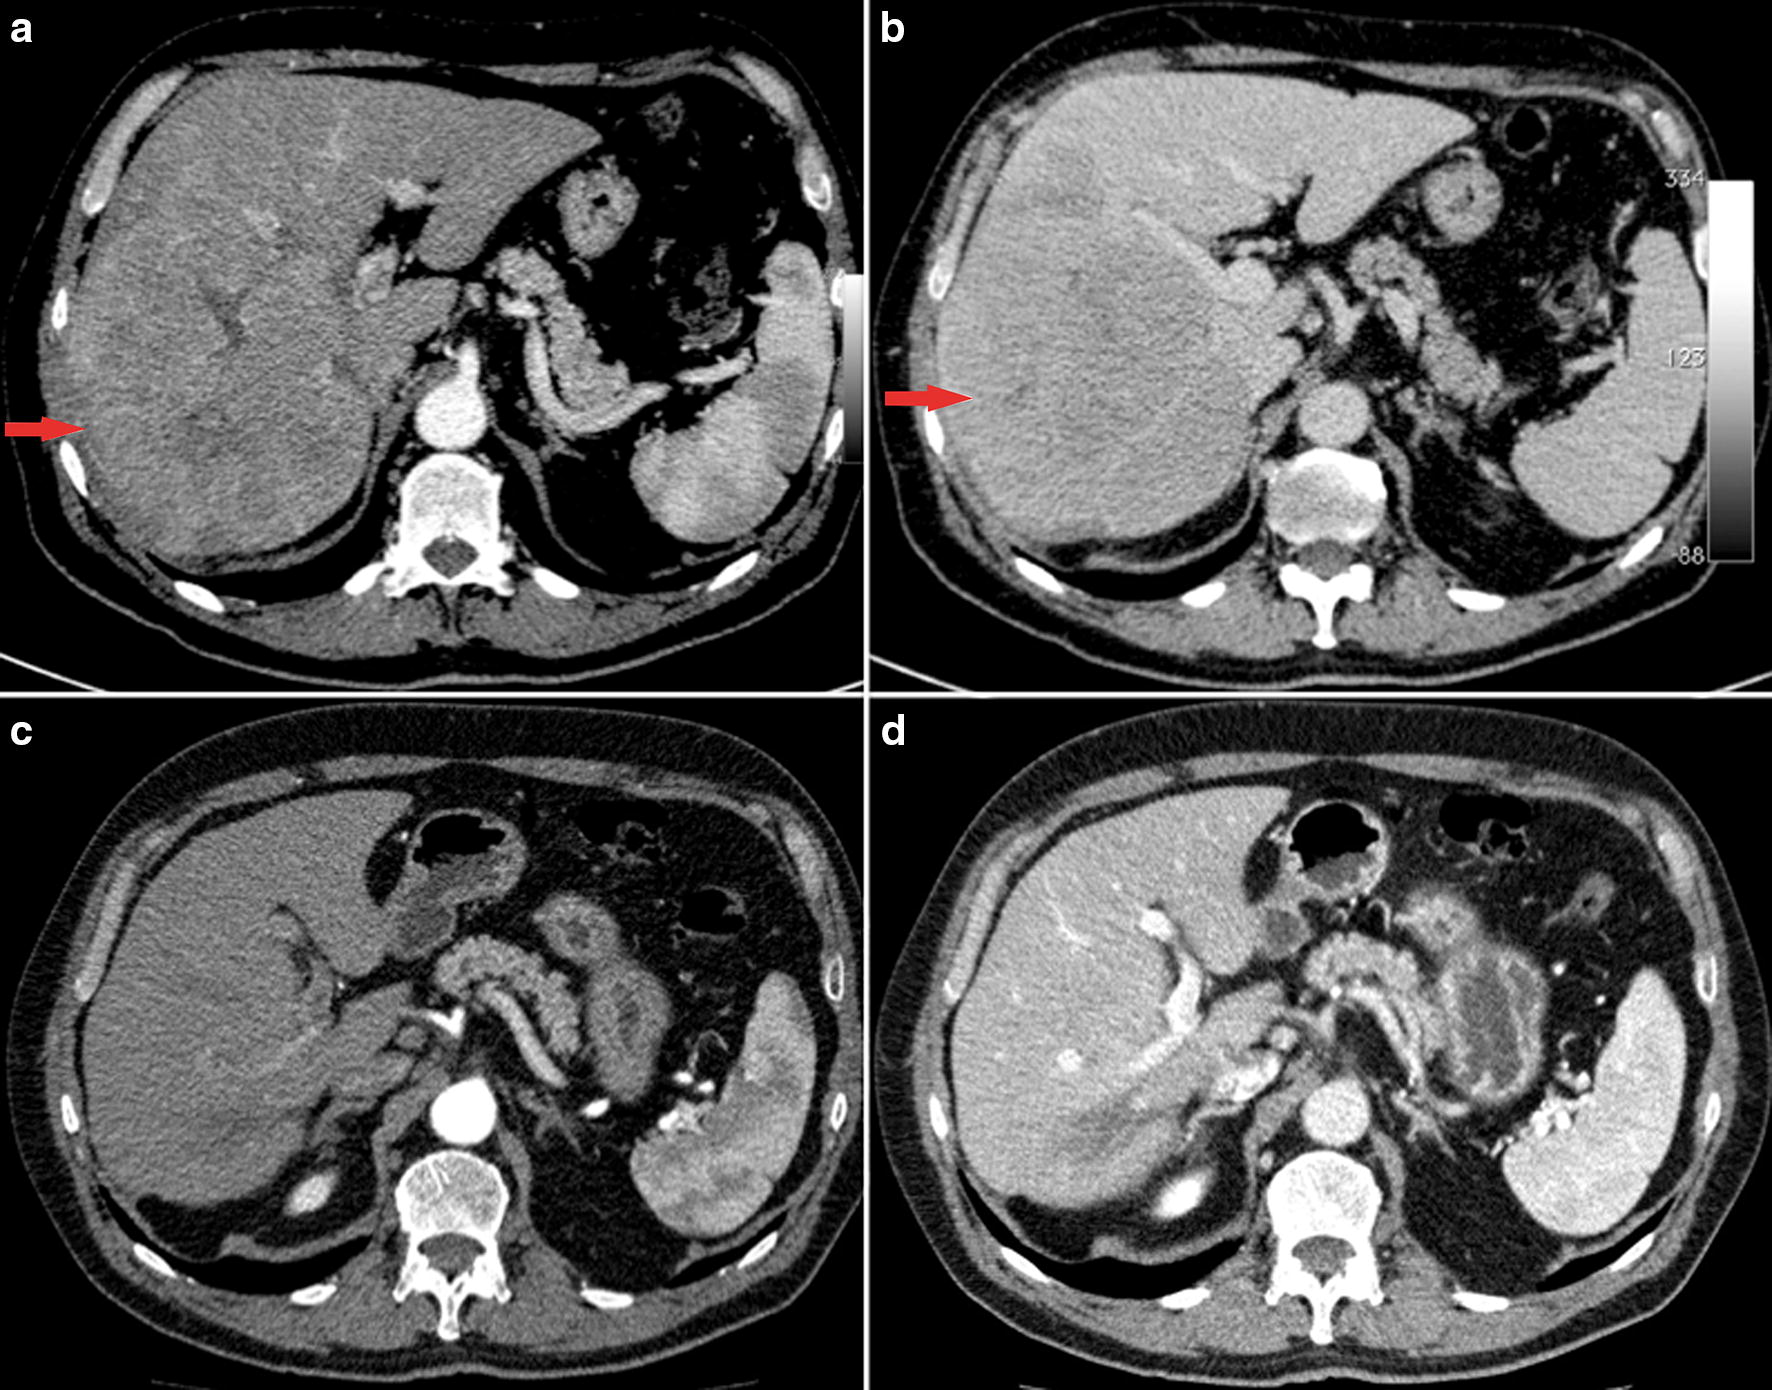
Lesions in case 2: axial CT images showing a large (10 cm) heterogeneous hypervascular hepatocellular carcinoma of segment VII in the arterial phase at baseline (a), with unequivocal wash-out in the venous phase (b); 32 months after starting systemic treatment with metronomic capecitabine, CT images show a complete response with the absence of viable tumour burden in the arterial phase (c), and only a 2 cm hypodense “scar” better identified in the venous phase (d)
